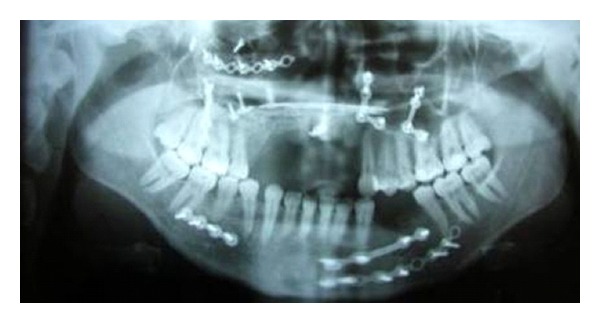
Panoramic radiograph showing mini plates and screws used for fixing fractured zygomatic arch, orbital, and maxillary sinus walls.
